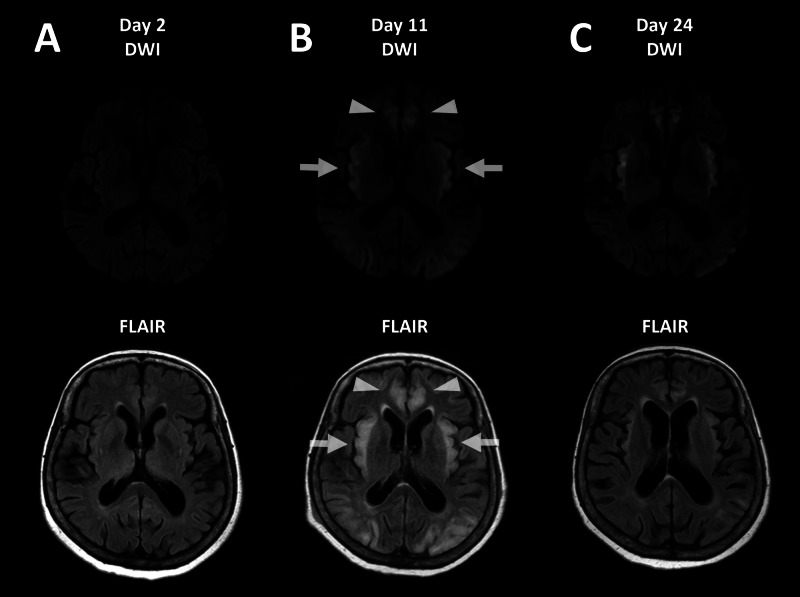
MR images of the brain (A) Initial MR images of the brain showing no remarkable changes. (B) MR images on day 11 showing symmetric abnormal signal intensity in the insular (arrows) and cingulate cortices (arrowheads) on diffusion-weighted imaging (DWI) and fluid-attenuated inversion recovery (FLAIR) imaging sequences. (C) MR images on day 24 showing improvement of the abnormal signal intensity.
